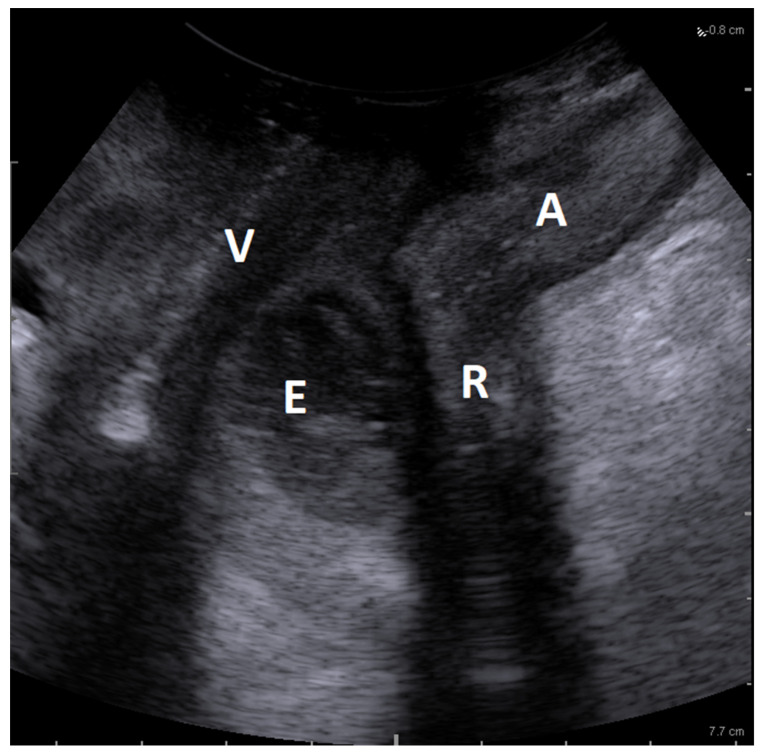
Translabial midsagittal sonographic view of the infected rectovaginal endometriosis, using a standard 2–5 MHz convex probe. Ultrasound evaluation showed an inhomogeneous iso-hypoechoic 3–4 cm lesion involving the rectovaginal septum, strictly connected to the rectal wall. V = vagina; A = anum; R = rectum; E = superinfected endometriosis.
